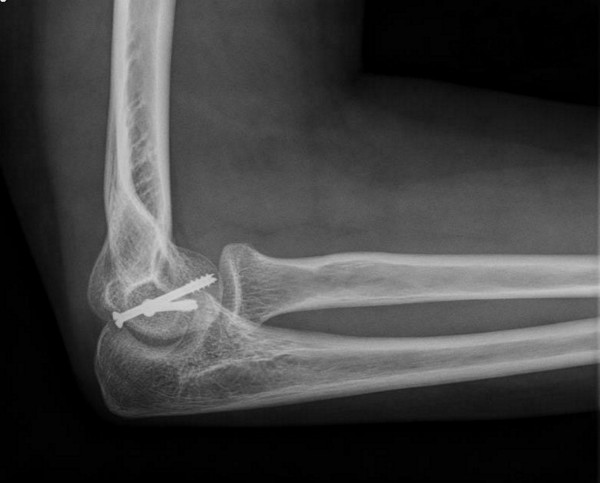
Lateral radiograph one year after the surgery, showing the fracture consolidation.
